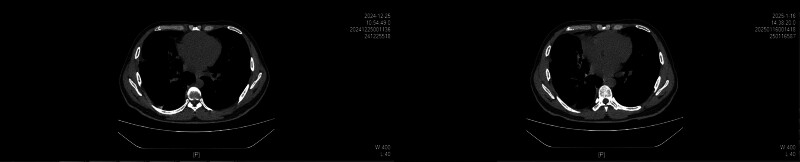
Chest CT scans showing no pleural effusion from 2 follow-up visits after discharge. CT = computed tomography.
